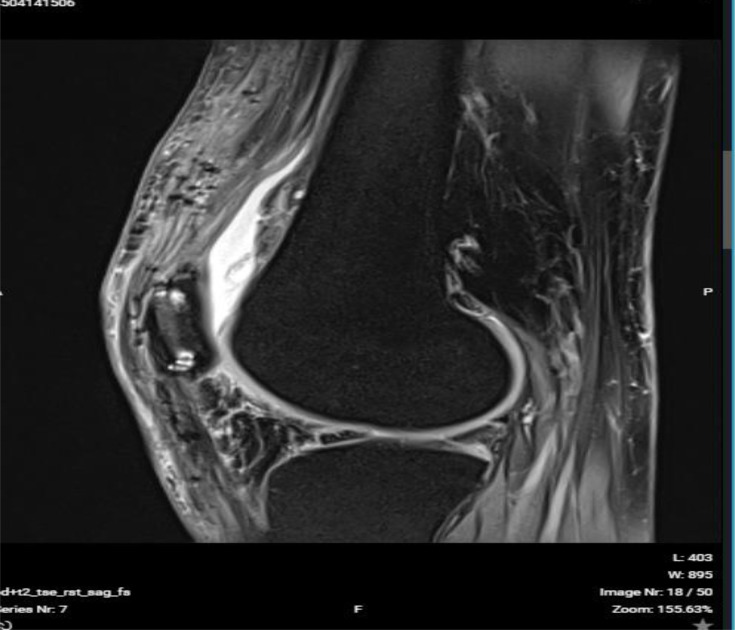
Post operative MRI: Notice the improvement of the patellar height and quadriceps fibrosis.
